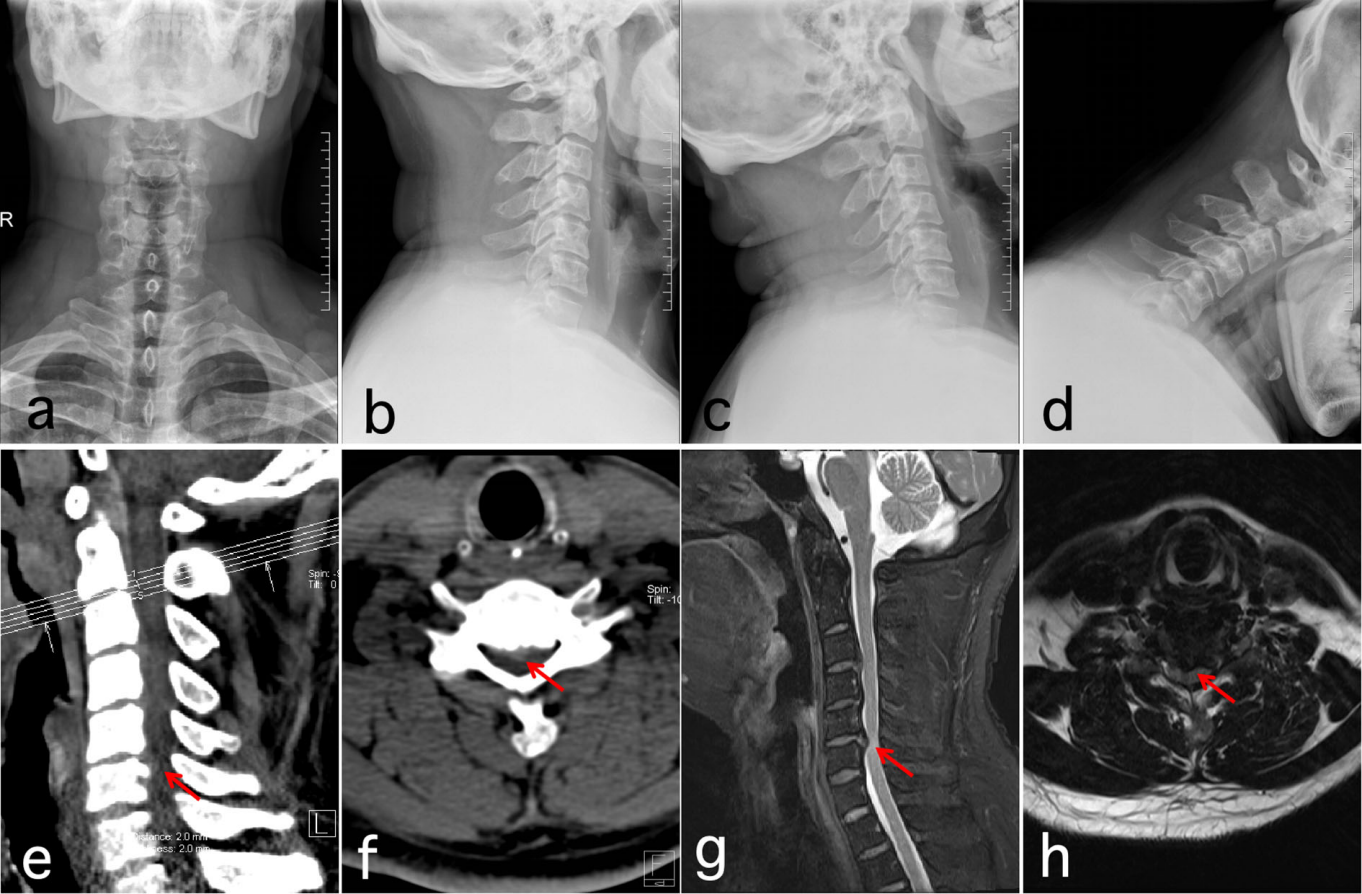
Images from a patient treated with Endo-ACDF for CSM. (a-d) Preoperative X-ray show cervical degeneration and straightening of the cervical physiological curvature; (e-h) Preoperative MRI and CT showed a herniated disc at the C5–6 segment with spinal stenosis and spinal cord compression.
